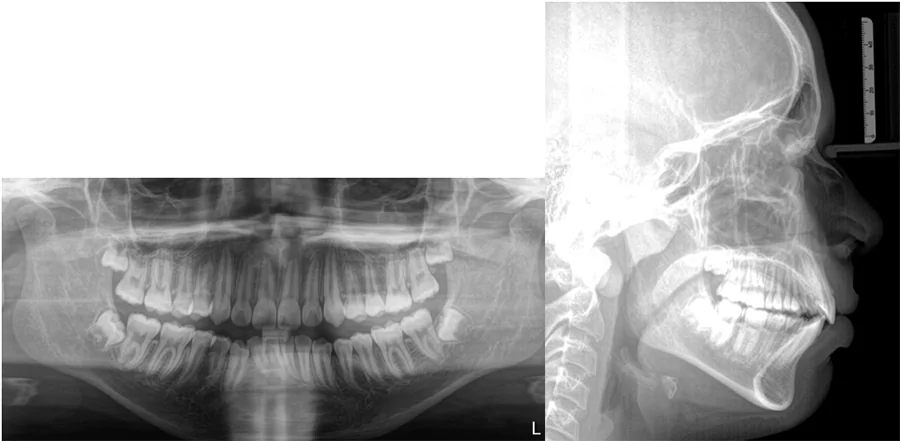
Control radiographs at the end of the functional orthopedic phase
